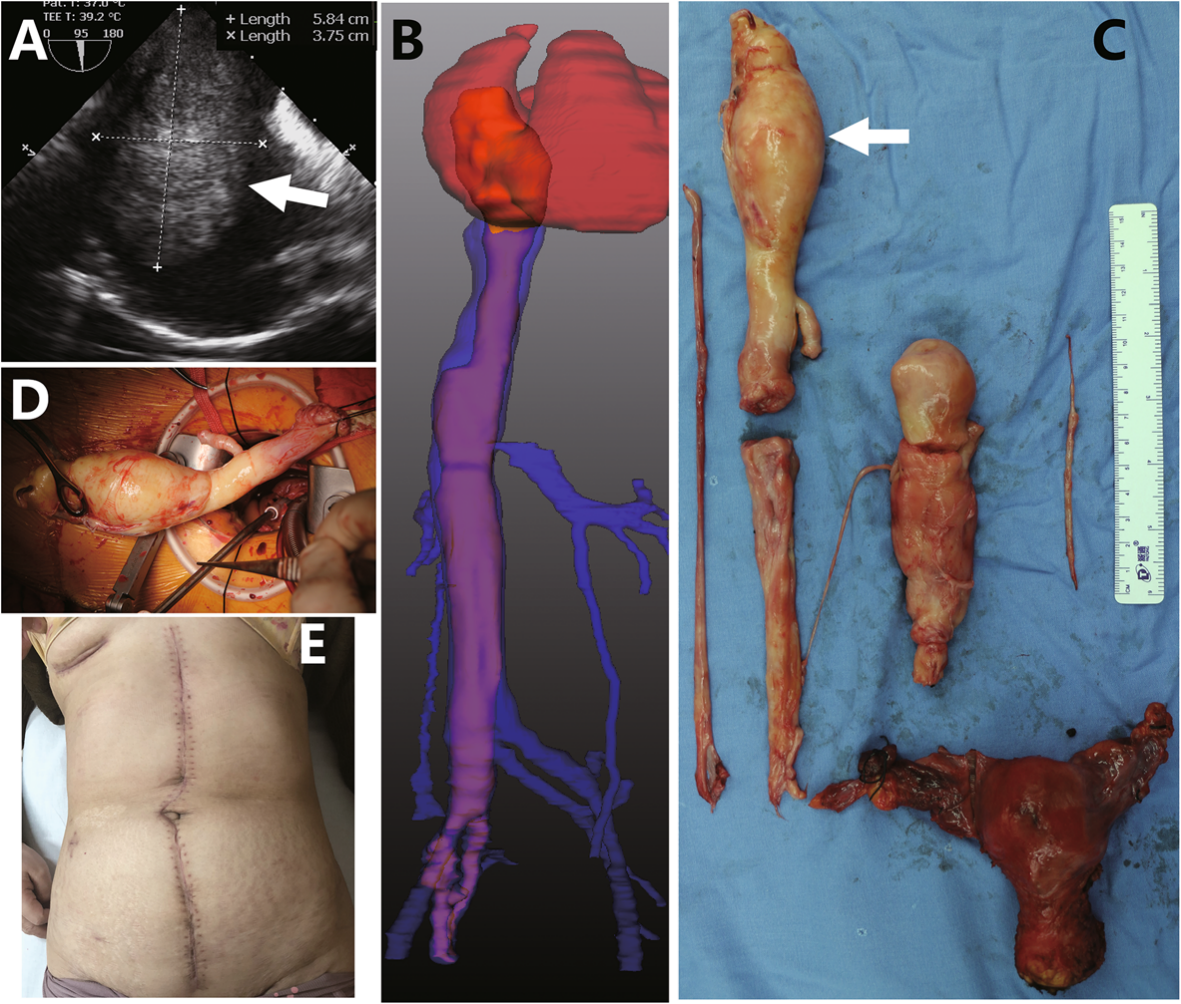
Echocardiogram (a) and 3D reconstruction of venography (b) indicate that the maximal diameter of intracardiac head is larger than the inlet of inferior vena cava. Tumor (c) is dissected through cavotomy and extracted upward through right mini-thoractomy (d, e). Arrow points to the maximal tumor dilation.
